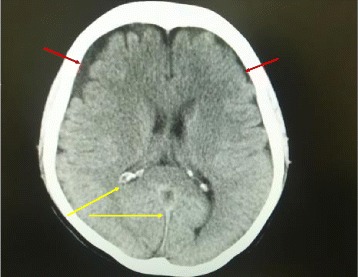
Computed tomogram obtained 9 days after the surgery. An increase in the size of the hematoma (red arrows) compared with that on the earlier MRI image is observed. A narrowed ventricle and midline shift (yellow arrows) are also observed, suggesting an increase in intracranial pressure
